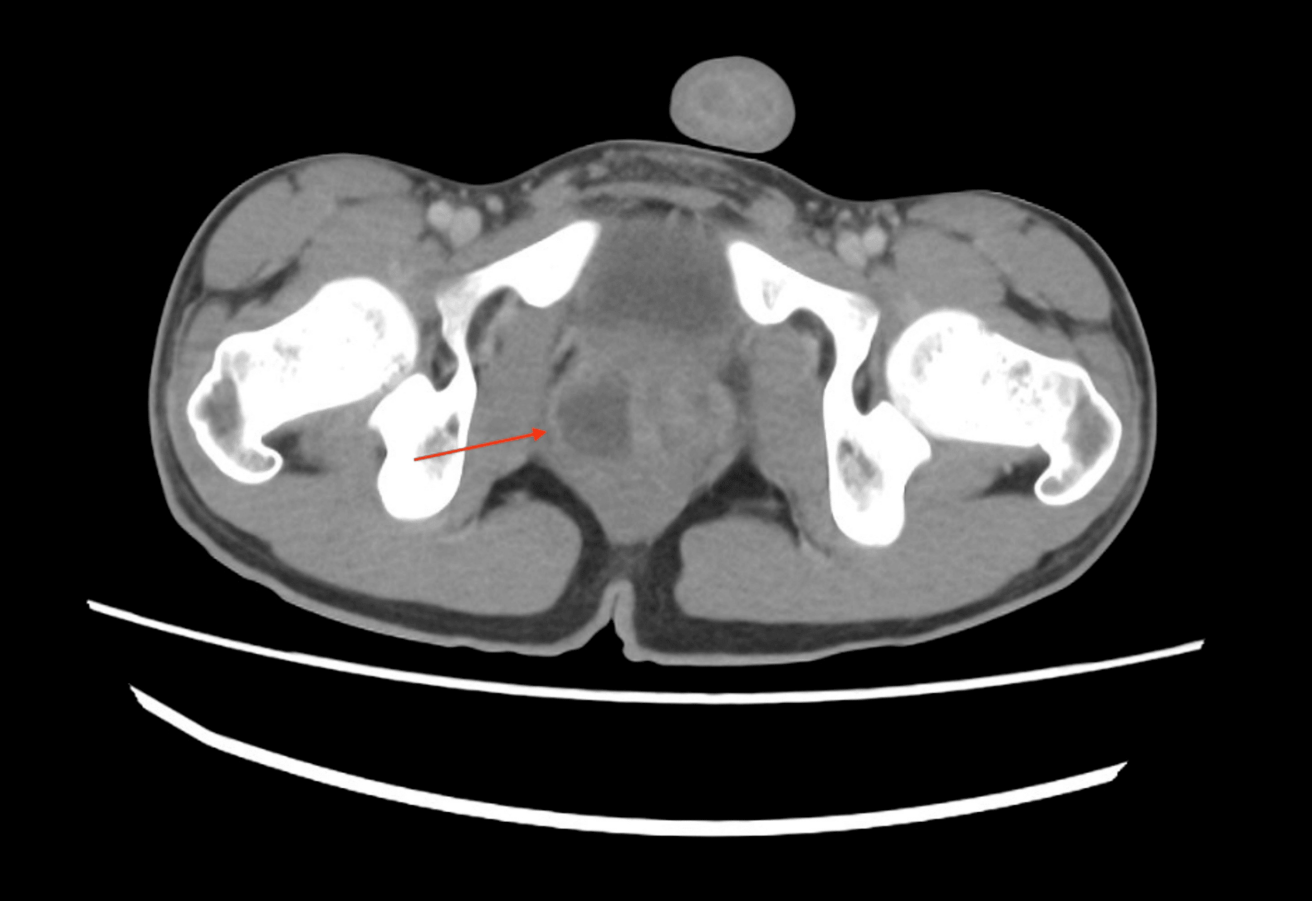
Contrast-enhanced CT scan of the pelvis in the axial view showing hypodense lesion in the right lobe of the prostate suggestive of prostate abscess CT: computed tomography
